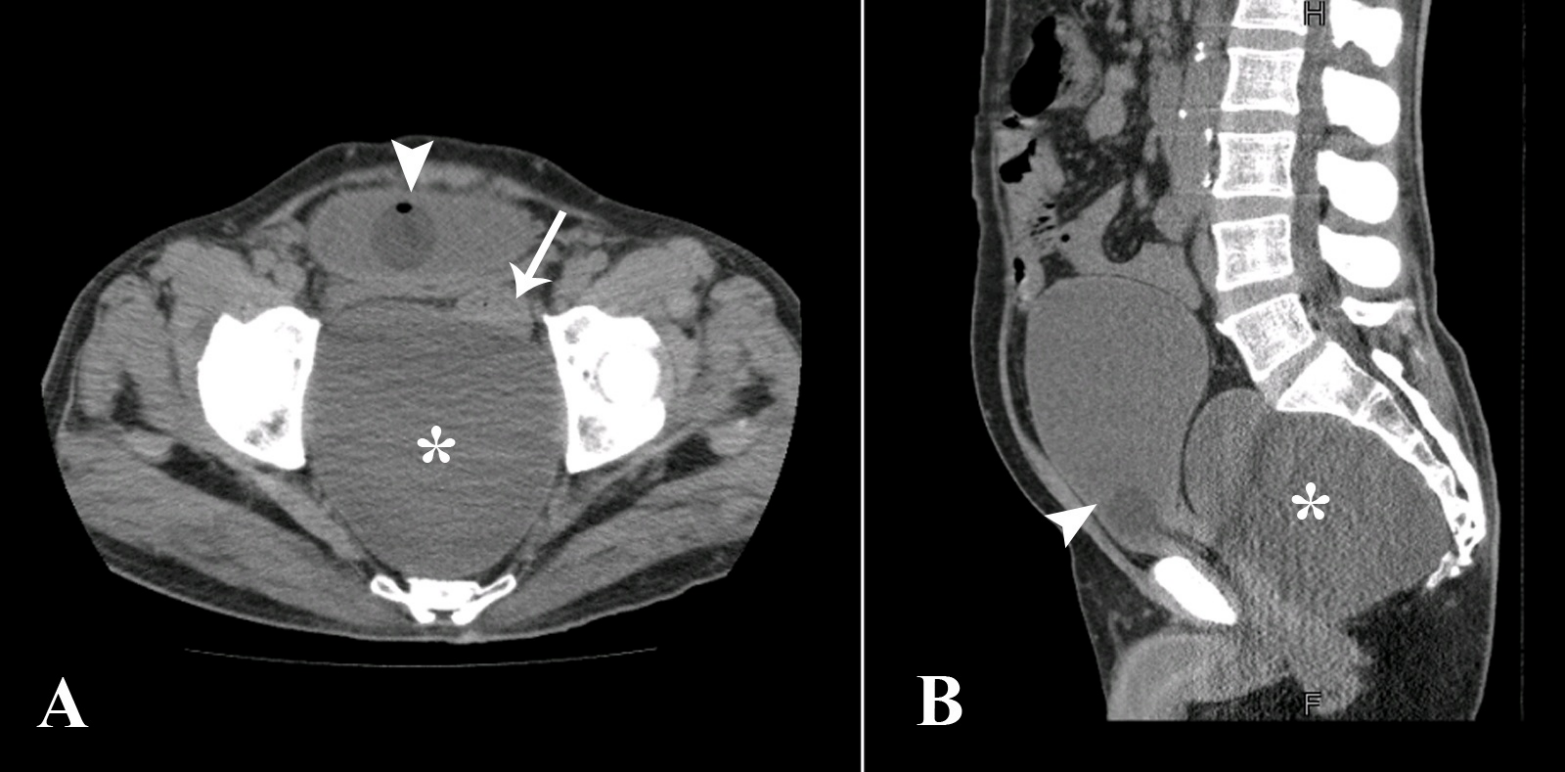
Axial (A) and sagittal (B) sections from CT-KUB A large well-defined low attenuation lesion in the presacral location (asterisk), displacing the urinary bladder superiorly and compressing the rectum in between (arrow). Foley’s catheter bulb can be seen in the bladder (arrowhead). CT-KUB: computed tomography scan of the kidneys, ureter and bladder
